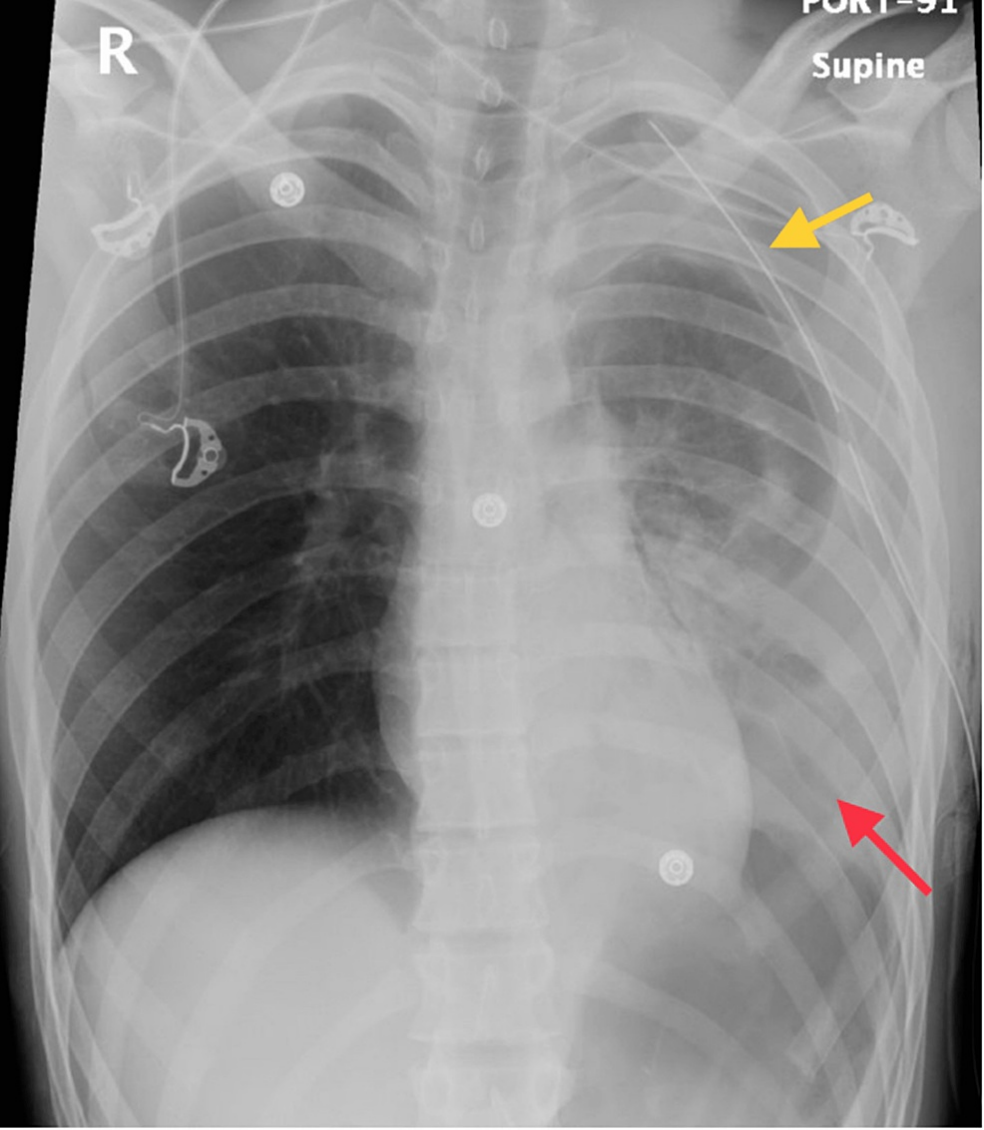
A repeated chest X-ray was performed to evaluate the position of the chest tube (yellow arrow). The image shows blood residuals on the left side (red arrow).
